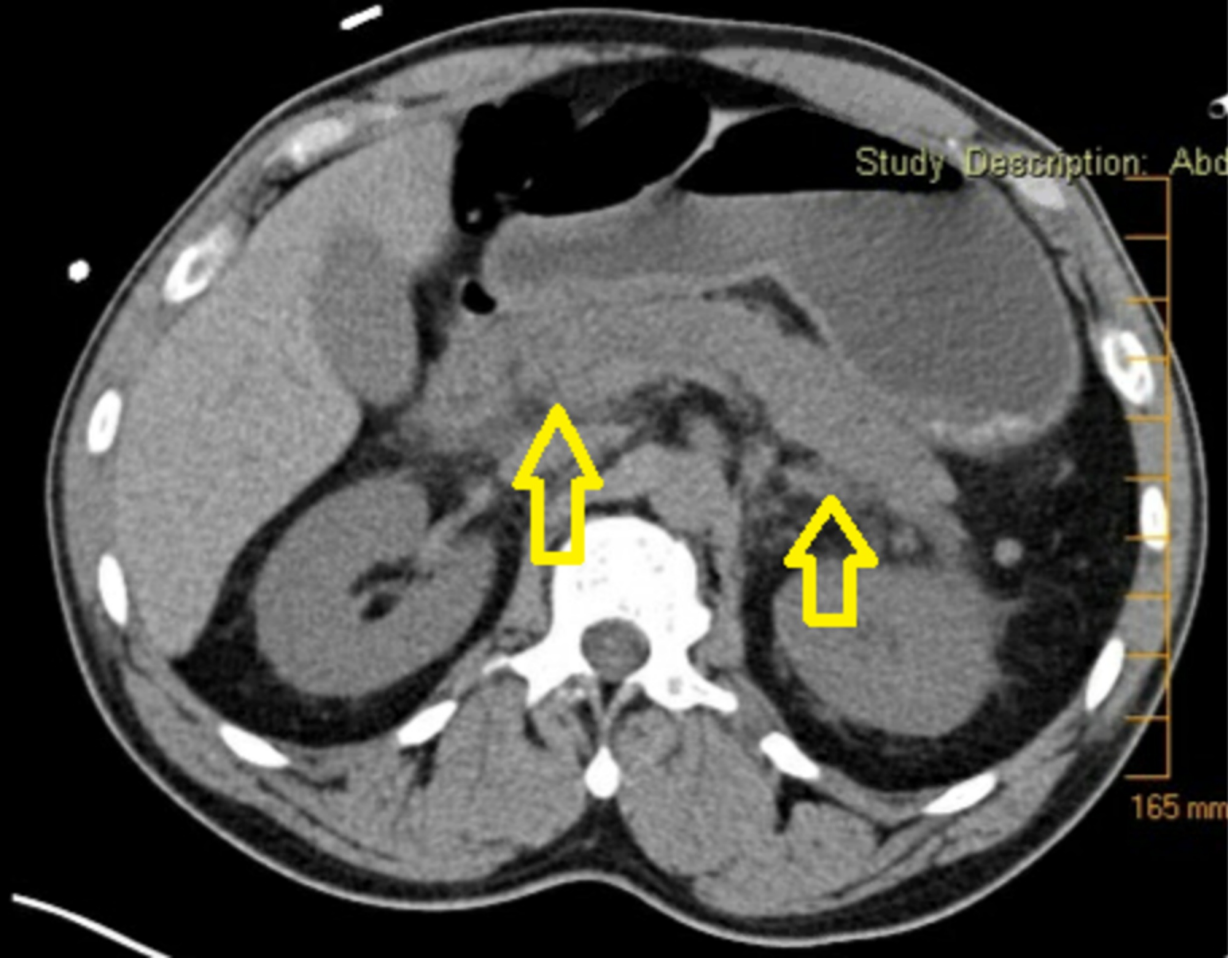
Computed tomography of the abdomen with arrows pointing towards the inflammation seen in pancreatitis
